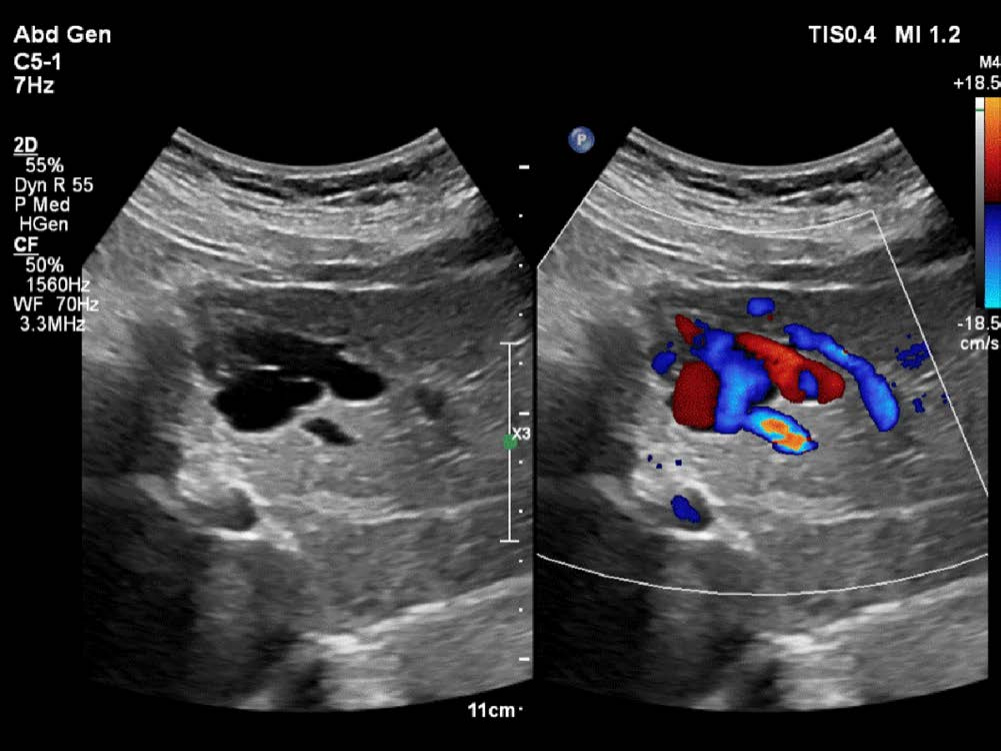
A color Doppler showed a cystic hepatic lesion with blood flowing through a channel to the medial branch of the left hepatic vein.
